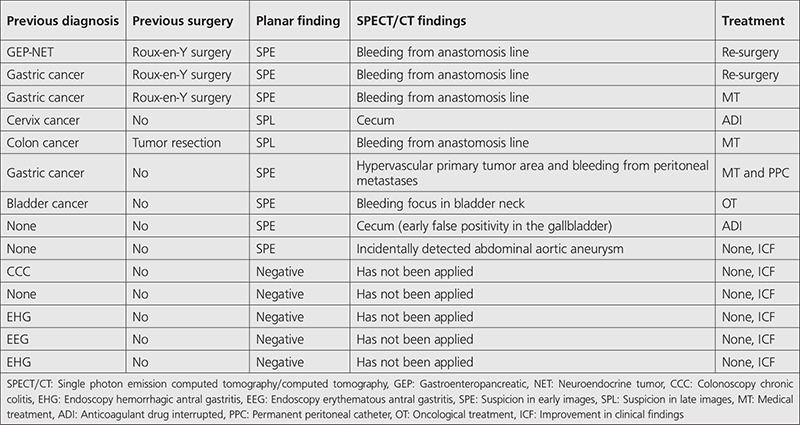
Clinical and scintigraphic characteristics of patients
